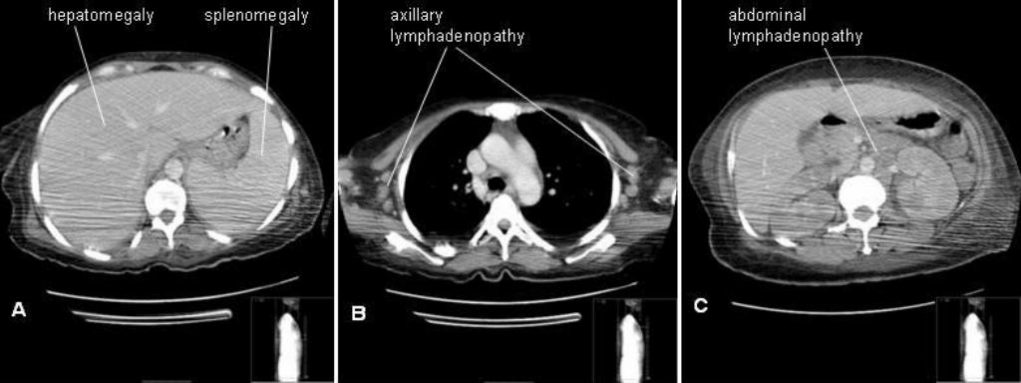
Multi-detector computed tomography (CT) thorax and abdomen with intravenous contrast enhancement showing hepatosplenomegaly, axillary and abdominal lymphadenopathy.
